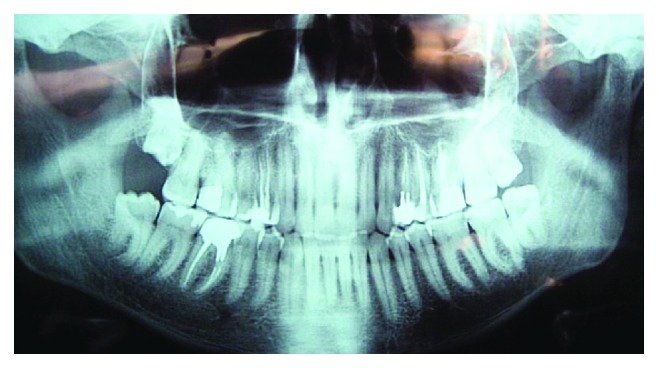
Orthopantomogram of patient K.
